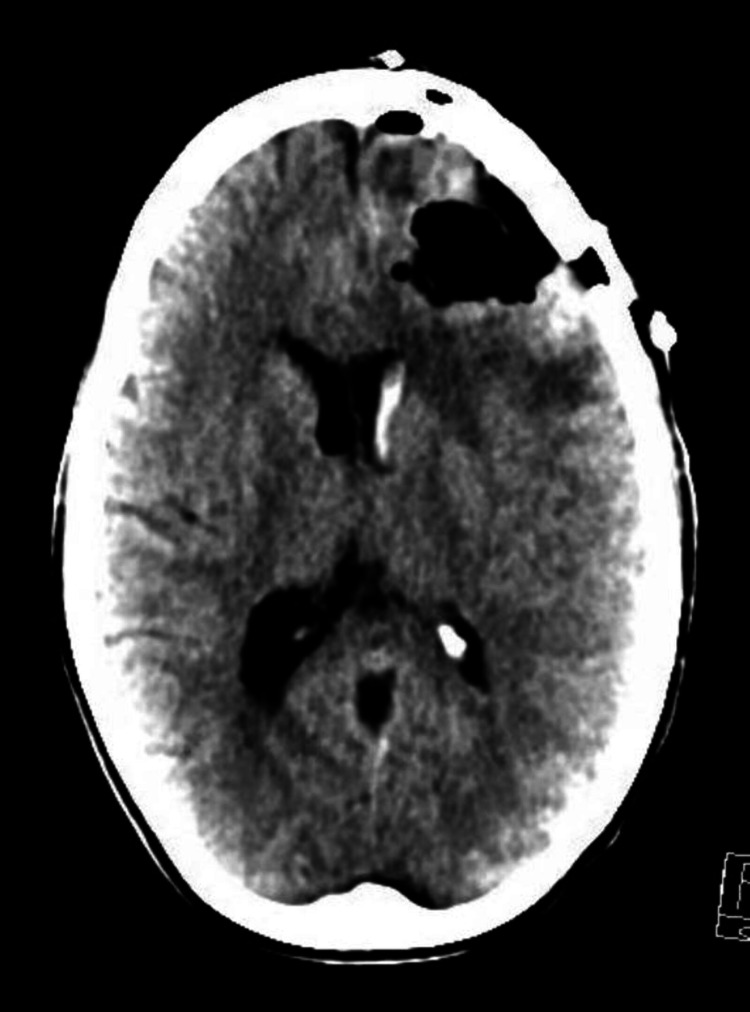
Noncontrast head CT in axial plane post surgical decompression showing a left frontal craniotomy defect from resection of left frontal hemorrhagic mass. There is postoperative hemorrhage and pneumocephalus within the surgical bed. Mass effect is significantly improved with resolution of left-to-right midline shift.
